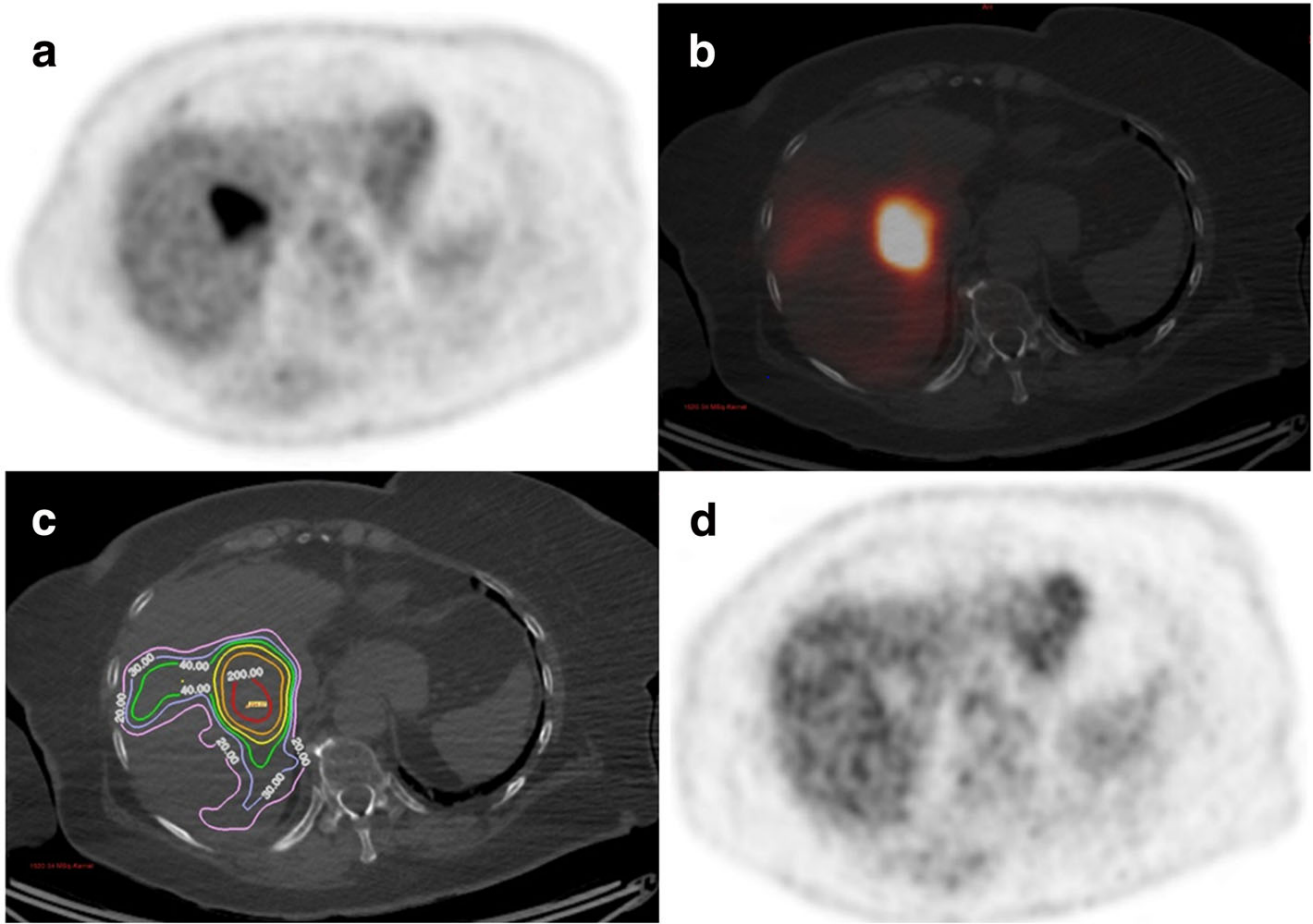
Registered axial images of a patient with liver mCRC. a Baseline FDG-PET shows the target lesion located in segment VIII. b
